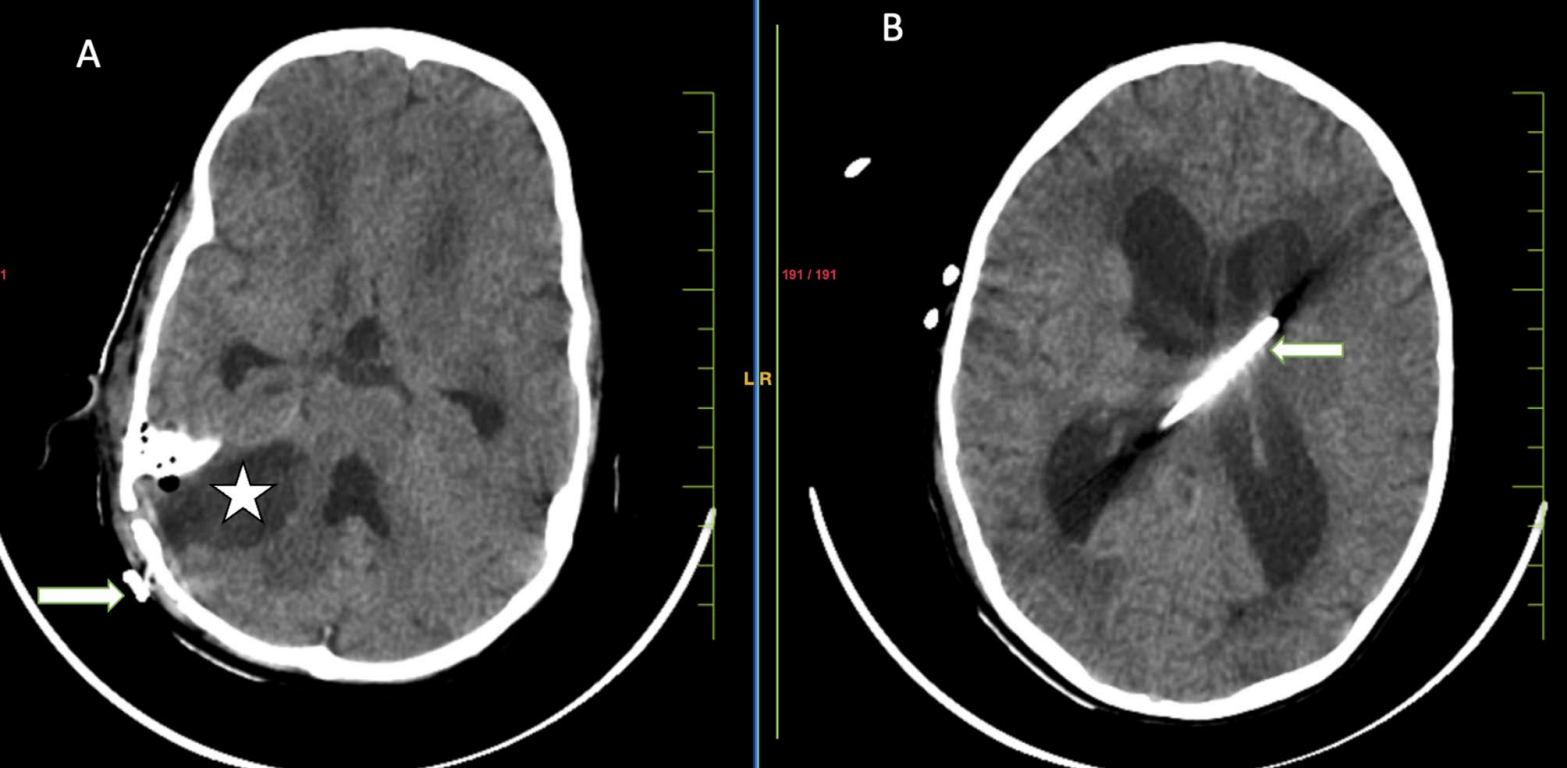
Post-operative non-contrast computed tomography (NCCT) brain. Axial CT images (A & B) showing right retromastoid suboccipital (RMSO) craniotomy defect with postoperative collection due to excised right cerebellar mass (★) and ventriculoperitoneal shunt (arrow)
